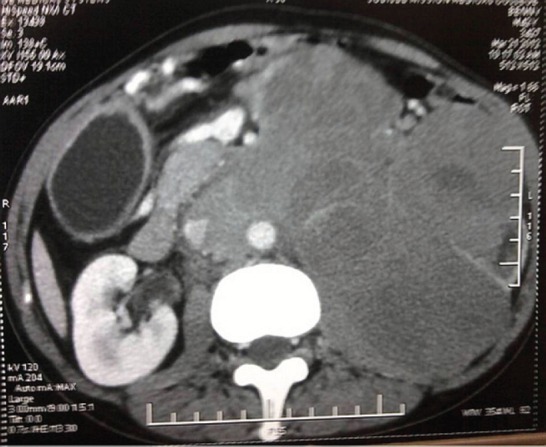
A contrast-enhanced CT of the abdomen showing large, lobulated homogenously enhancing mass lesion 14×12×18 cm arising from left kidney and encasing the renal vessels.
